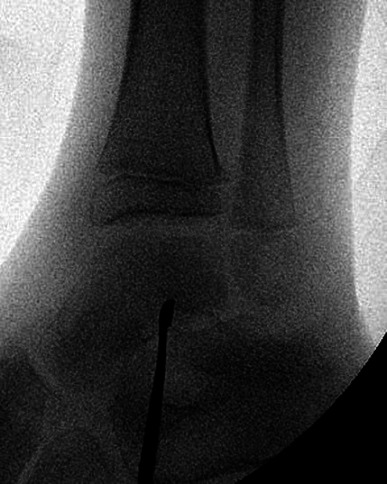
An intra-operative radiograph showing the position of the curette within the sub-talar space.
